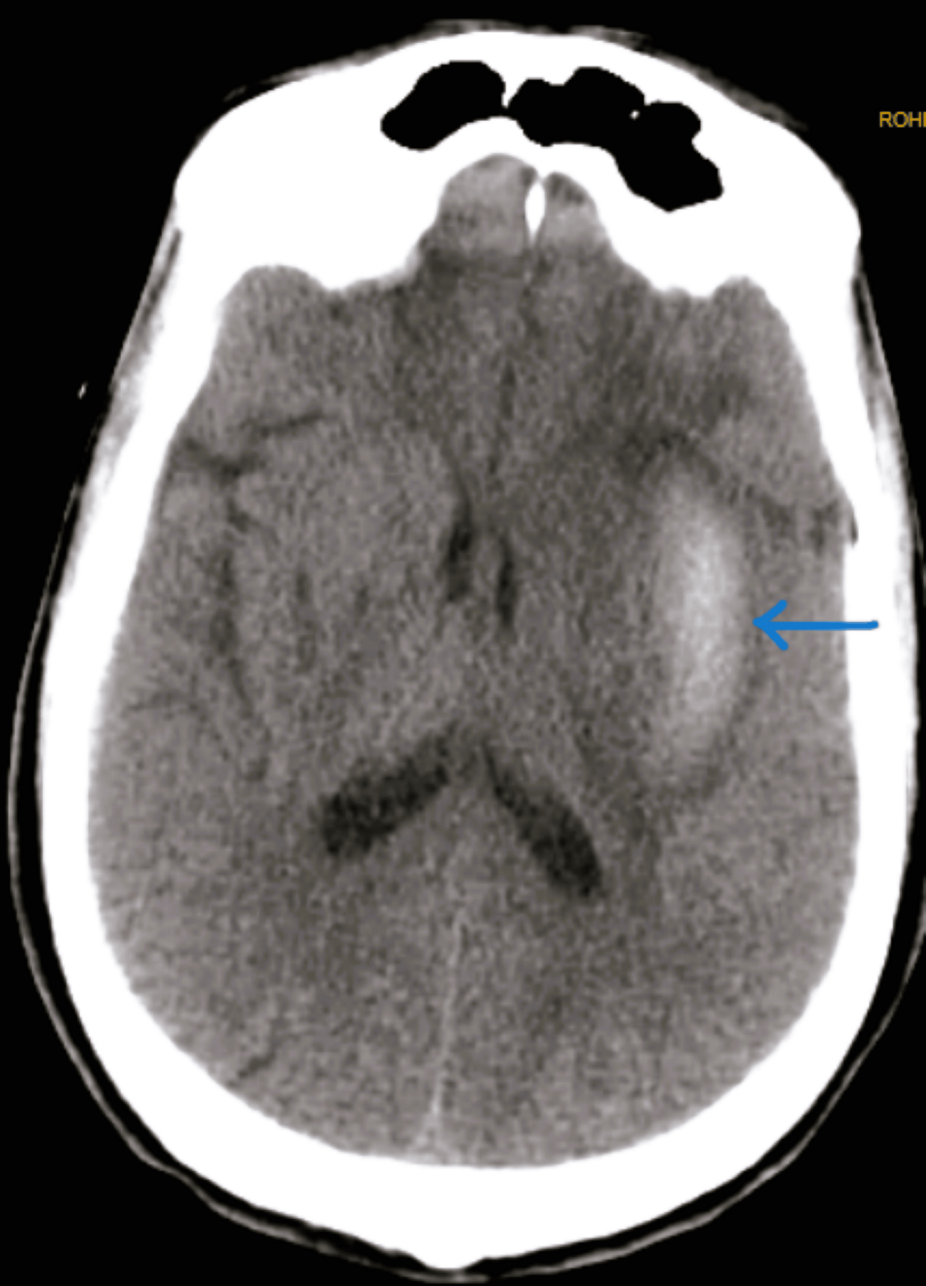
CT brain axial section showing an intraparenchymal hemorrhage (arrow) in the left external capsule
